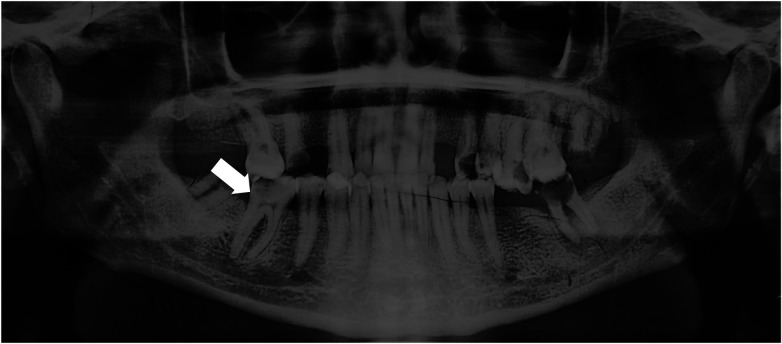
Preoperative panoramic radiograph showing a coronal radiolucency extending to the inner third of dentin in tooth 46. Note the close anatomical relationship between the roots and the inferior alveolar nerve canal (distance <4 mm).
